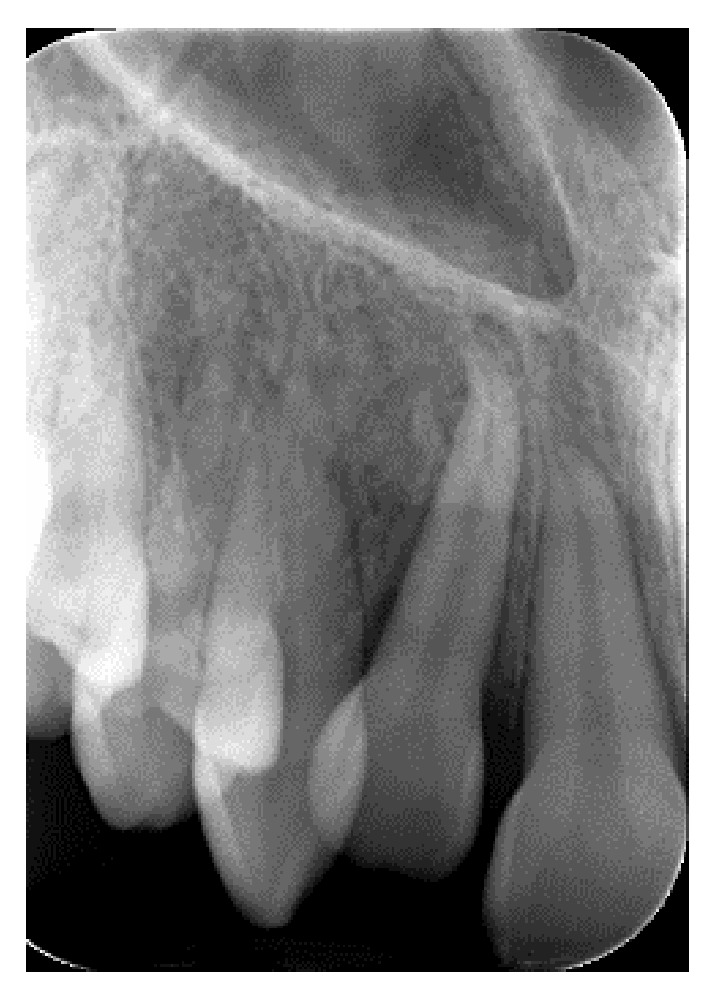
Periapical radiograph after traumatic injury.
